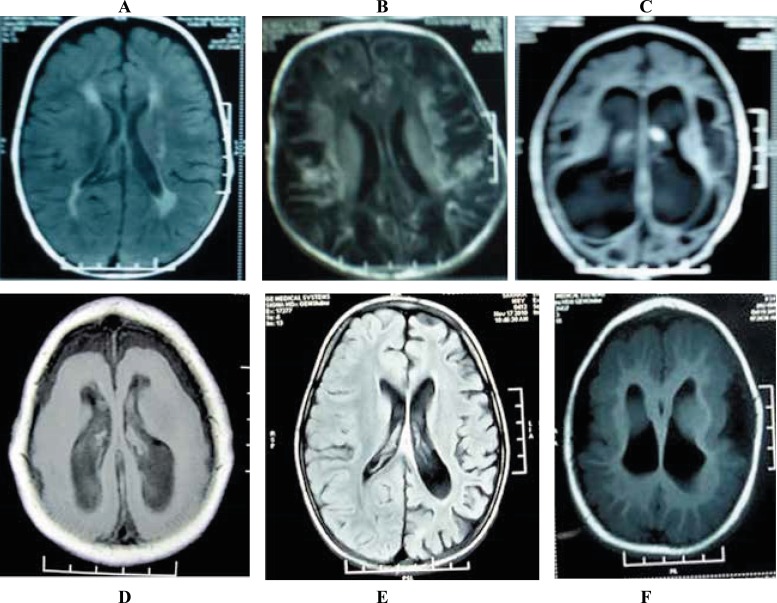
MRI findings of the studied children: A) Flair image axial section showing periventricular leukomalacia (hyperintensities around ventricles and peritrigonal areas), B) Flair image axial section showing cystic encephalomalacia (multiple cystic lesions and gliotic changes, and loss of grey and white matter). C) Flair image axial section showing porencephalic Cyst, D) T1-weighted image axial section showing lissencephaly (loss of sulci and gyri, smooth counter of both cerebral hemispheres), E) Hemiatrophy-flair image axial section showing cortical atrophy in left cerebral hemisphere and ex- vacuo dilatation of ventricles, F) T1-weighted image axial section showing pachygyria-thickening of cortical matter
